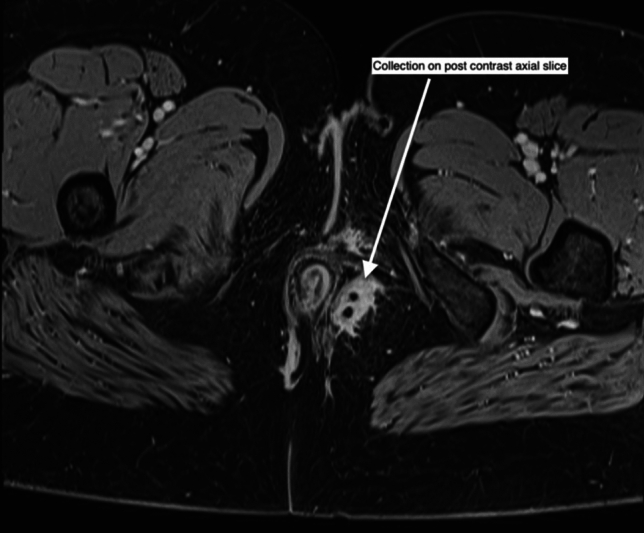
Collection on postcontrast MRI axial slice
